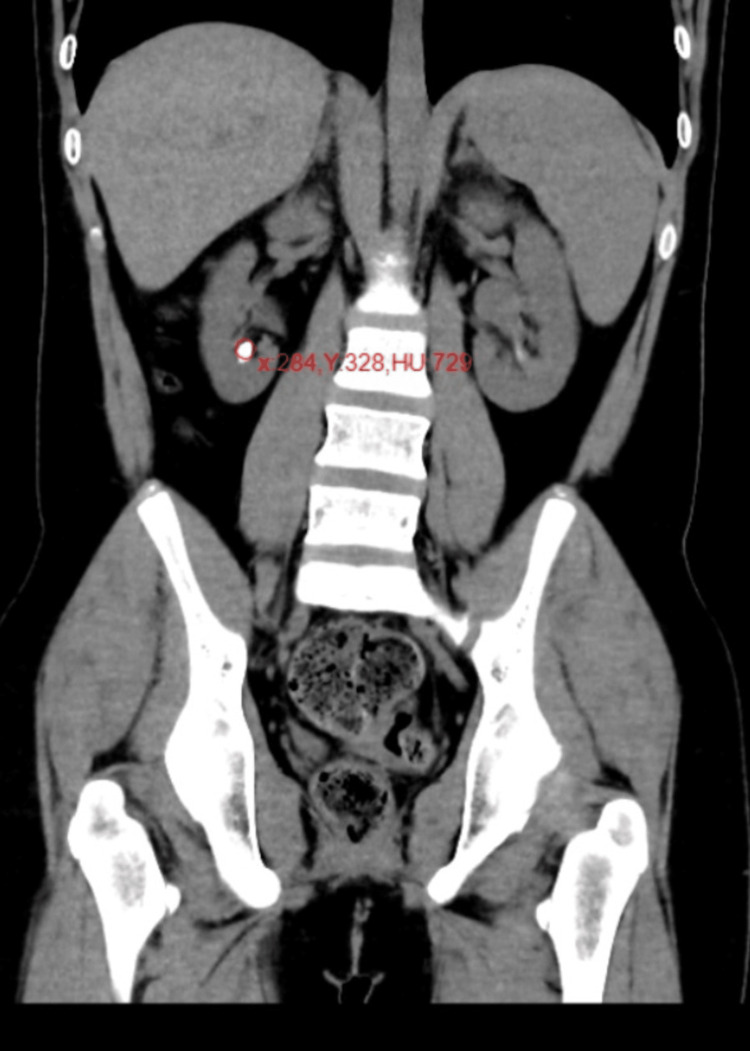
CT scan showing a right lower calyceal stone measuring 10 × 6 mm, with a CT attenuation of approximately 742 Hounsfield units (HU). Although non-contrast CT is the standard imaging modality for urolithiasis, contrast-enhanced CT was performed in this patient to better delineate the complex renal collecting system anatomy, given the known duplex ureter and the extensive history of prior urological interventions [2]. Contrast imaging aided in procedural planning and the assessment of the collecting system prior to management.
